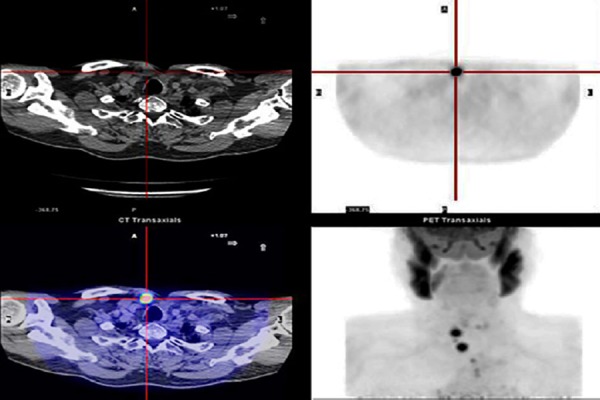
Images of a 65-year-old male patient with papillary thyroid cancer undergoing total thyroidectomy and ^131^I therapy with a total dose of 550 mCi. ^131^I WBS after the third treatment session showed no abnormal uptake and the patient’s stimulated thyroglobulin was > 1000 ng/ml. (B) axial PET and (C) axial PET/CT images revealed increased focal ^18^F-FDG uptake in thyroid bed and pretracheal lymph node, which is not clearly seen in low-dose CT (A). Surgical resection of these lesions confirmed recurrence/metastasis of papillary thyroid carcinoma
